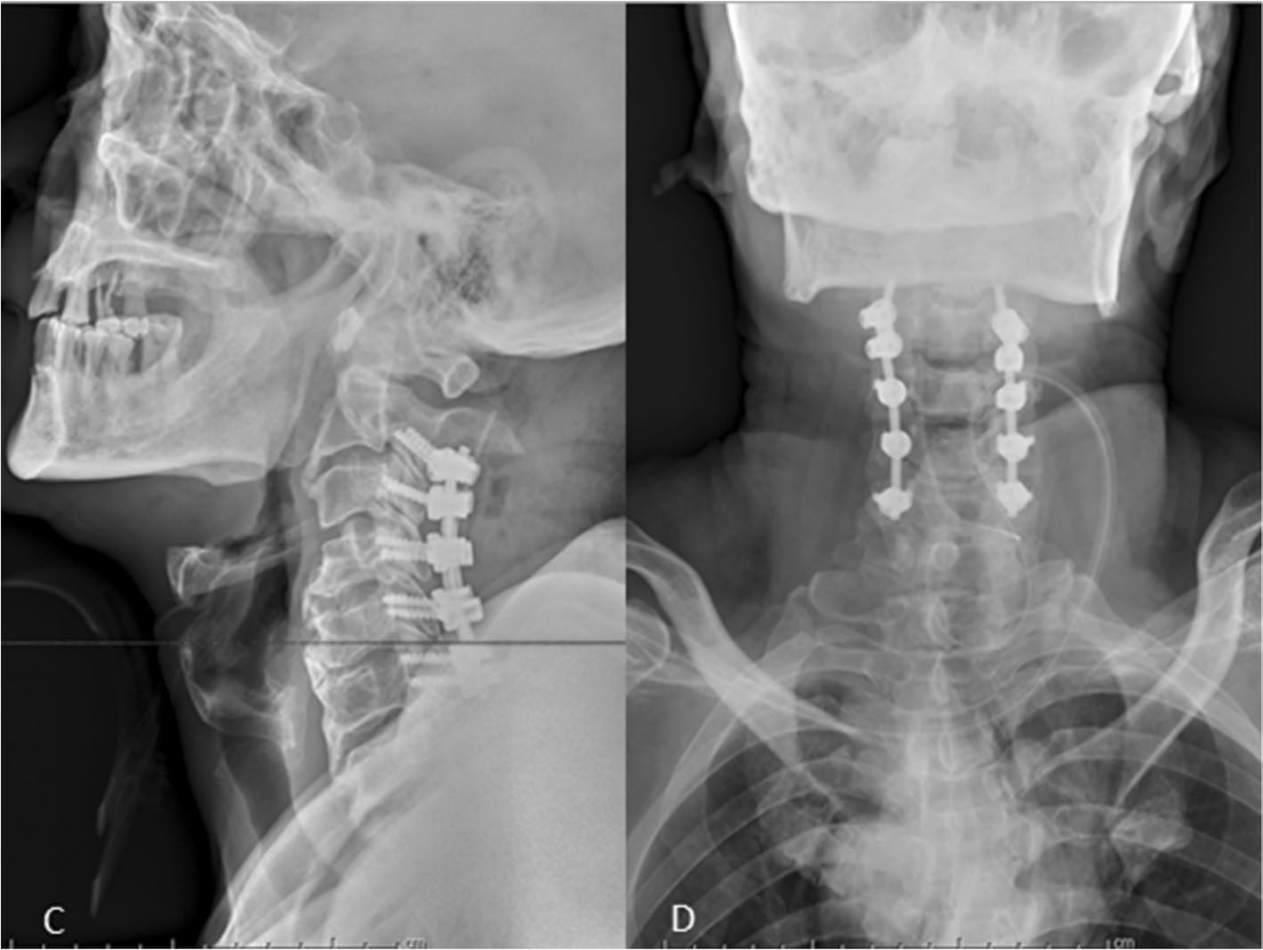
C Lateral and D anterior X-rays show that pars screws were inserted into C2
